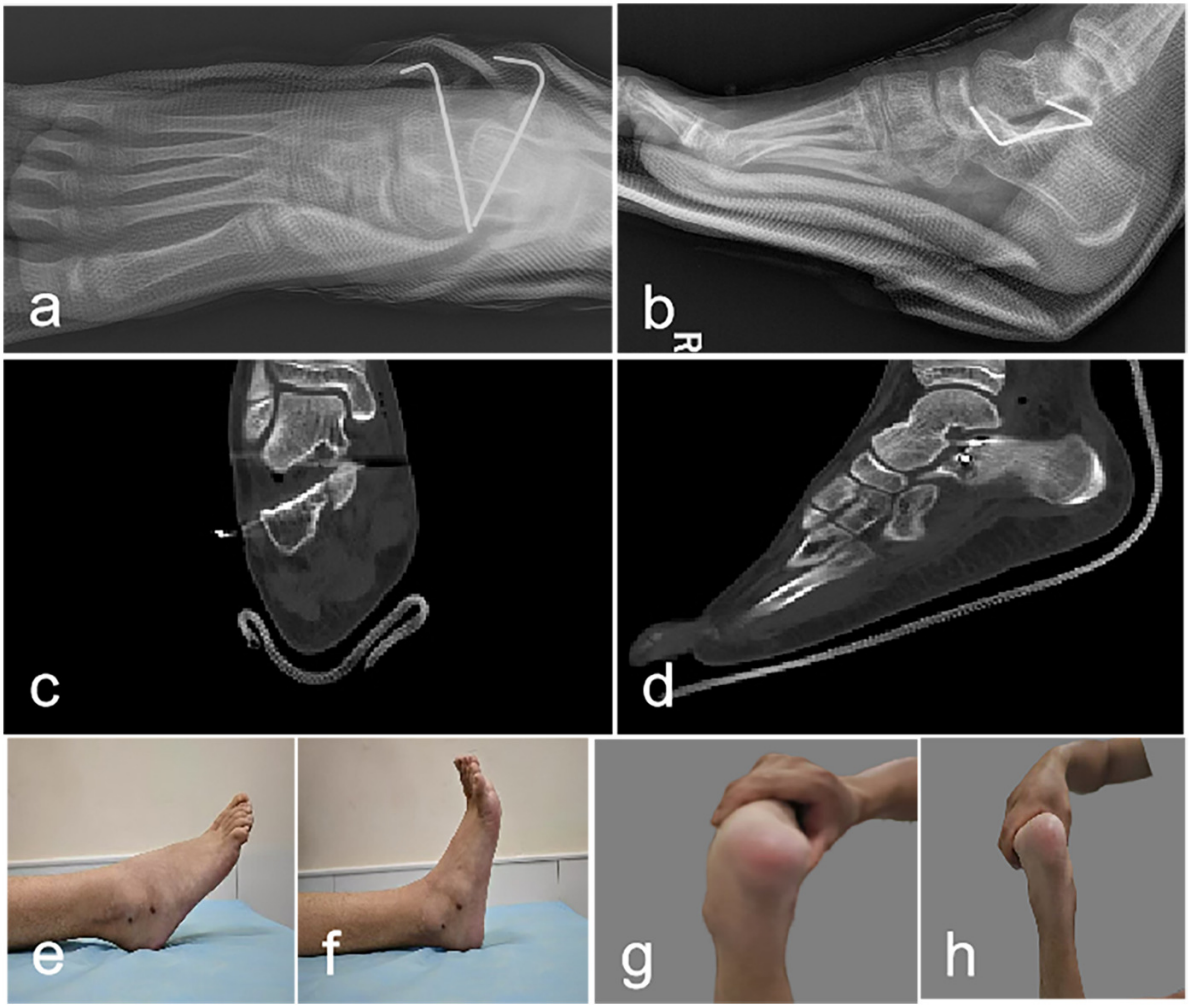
Postoperative x-ray, CT images, and ankle joint range of motion. (a,b): Postoperative radiographic imaging demonstrates adequate fracture reduction with acceptable anatomical alignment; internal fixation hardware maintains stable positioning. (c,d) Coronal and Sagittal CT image demonstrates smooth articular surfaces. (e–h): Three-month follow-up examination revealed normal plantarflexion, inversion, and eversion with mild dorsiflexion limitation.
